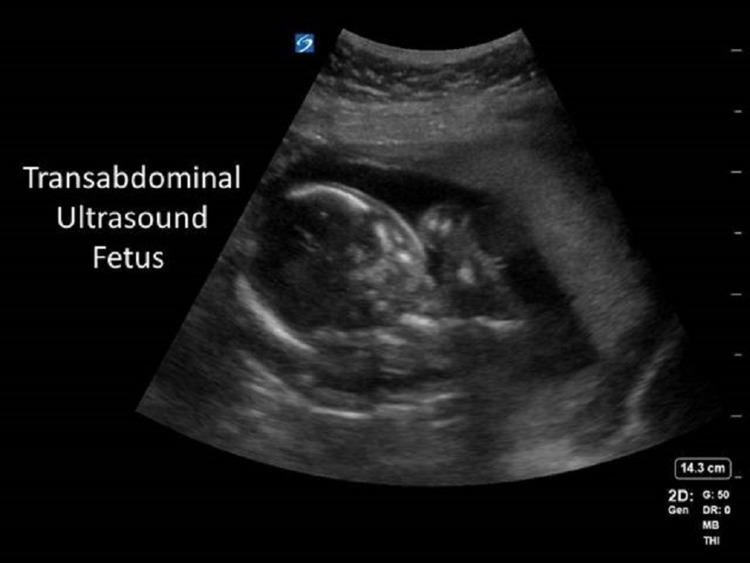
Representative transabdominal ultrasound fetus.
